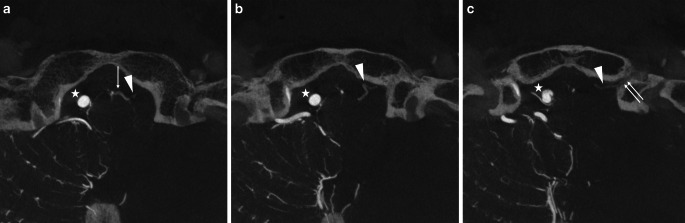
A representative case of the anterior condylar vein (ACV) bridging vein (BV). Axial view of the slab MIP images from contrast-enhanced cone-beam CT shows that the transverse medullary vein (*arrow* in (a)) drains into the ACV (*double arrows* in (c)) in the hypoglossal canal via the ACV BV (*arrowheads* in (a)–(c)). *Star* shows the right vertebral artery
